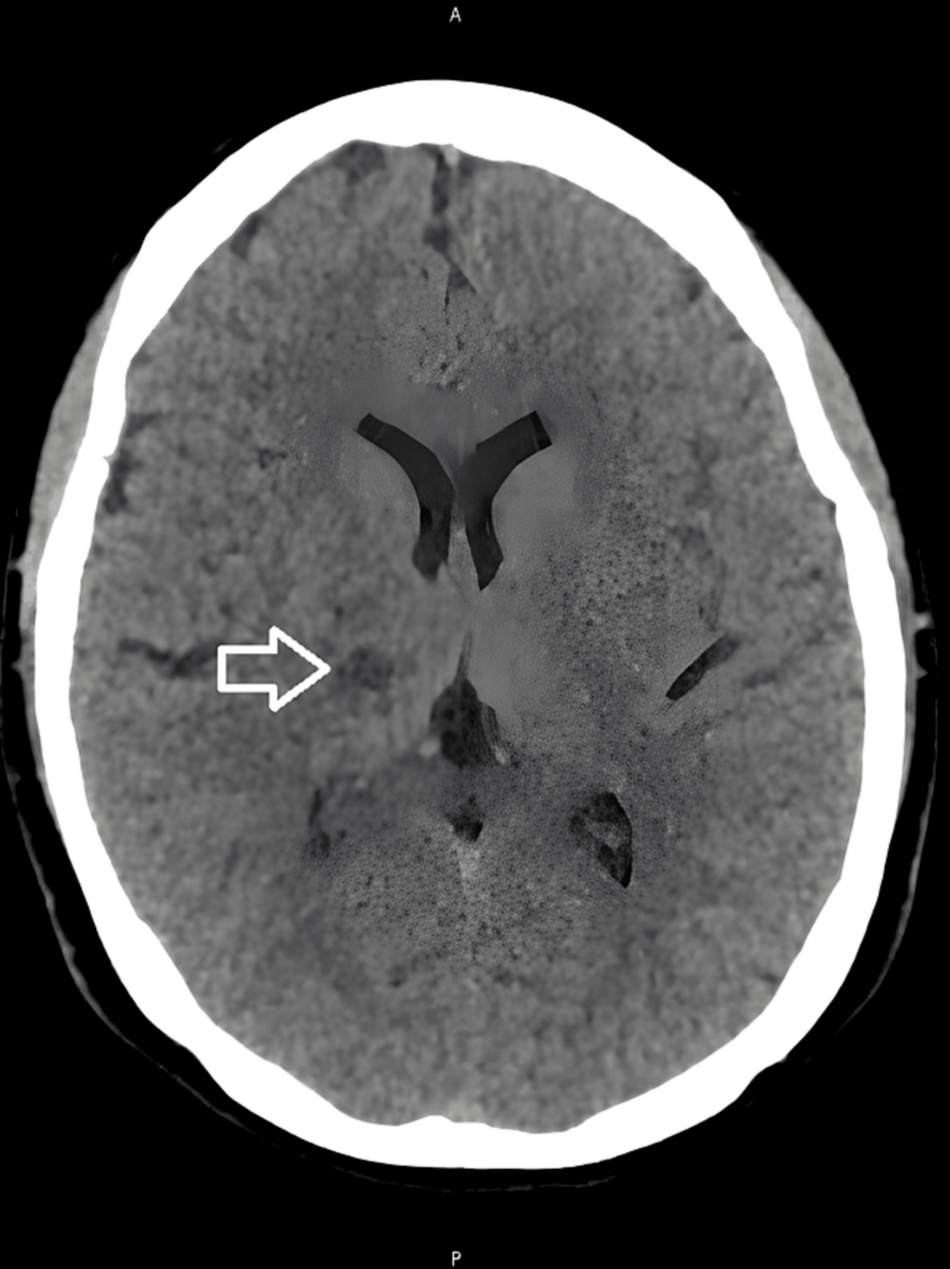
A plain CT head showing a focal hypodensity in the right capsulothalamic region suggestive of a recent infarct.
